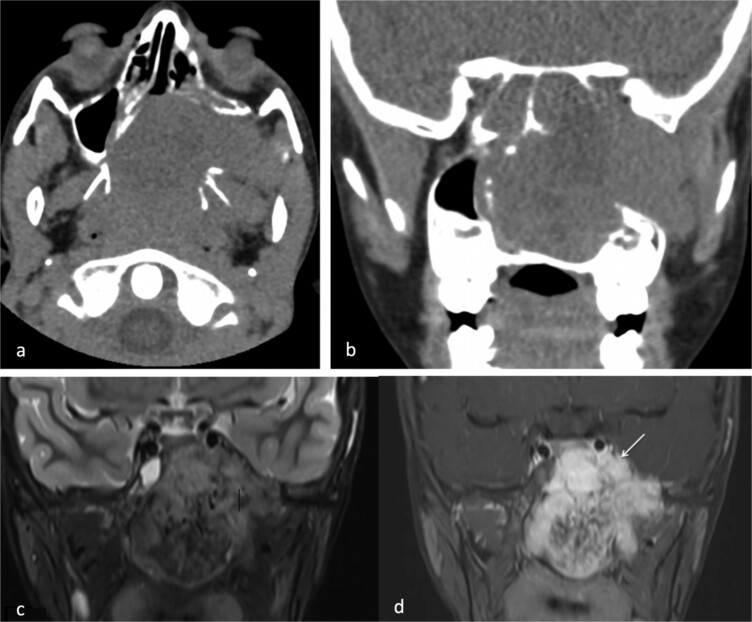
(a) Axial non-contrast CT of the paranasal sinuses showing a soft tissue mass obliterating the posterior aspect of the nasal cavity, with extension and remodeling of the left pterygopalatine fossa into the infratemporal fossa. (b) Coronal image demonstrating superior extension into the left sphenoid sinus and left inferior orbital fissure. (c) Coronal T2-weighted MRI showing a large JNA with heterogeneous signal intensity and multiple flow voids consistent with hypervascularity. (d) Post-contrast coronal T1-weighted MRI demonstrating avid contrast enhancement and invasion of the left Meckel’s cave and cavernous sinus (arrow).
